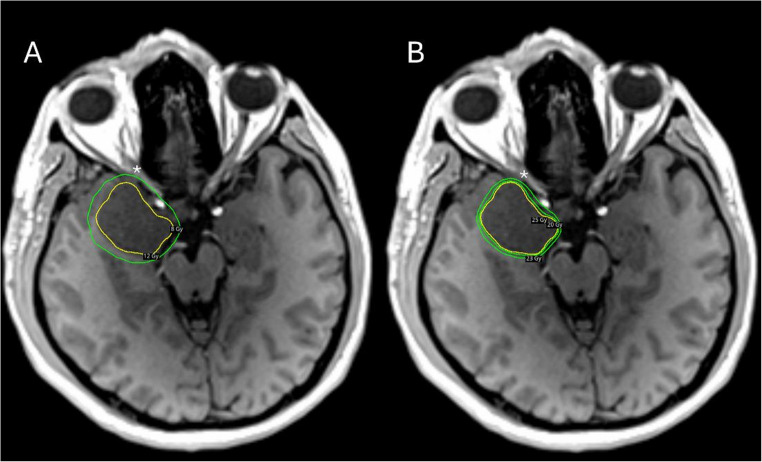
Comparative dose distribution on axial non-contrast T1-weighted MRI (selected for optimal optic nerve visualization) in a representative case (Case 2). (A) Hypothetical single-fraction plan delivering 12 Gy marginal dose: the 8 Gy isodose line (green), which represents the widely accepted single-fraction tolerance threshold for the optic apparatus (asterisk), extends to encompass the ipsilateral optic nerve, indicating that safe single-fraction treatment would not be feasible for this large, optic-adjacent lesion. (B) Actual hypofractionated plan delivering 25 Gy in five fractions: the prescription isodose (25 Gy, yellow) provides conformal target coverage, while the 23 Gy and 20 Gy (green, outer) isodose lines remain well clear of the optic nerve (asterisk), demonstrating effective sparing of the anterior visual pathway. The five-fraction tolerance limit for the optic apparatus (25 Gy) is not reached at the optic nerve in this plan, confirming the dosimetric advantage of the hypofractionated approach
